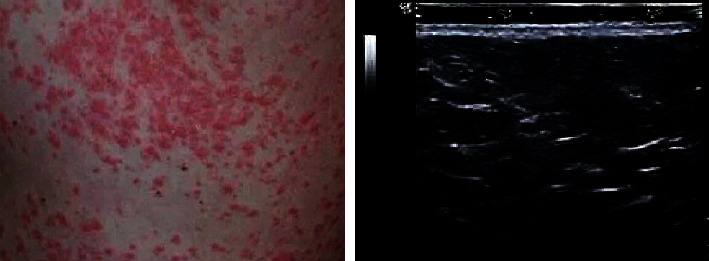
Psoriasis skin and ultrasound images.
